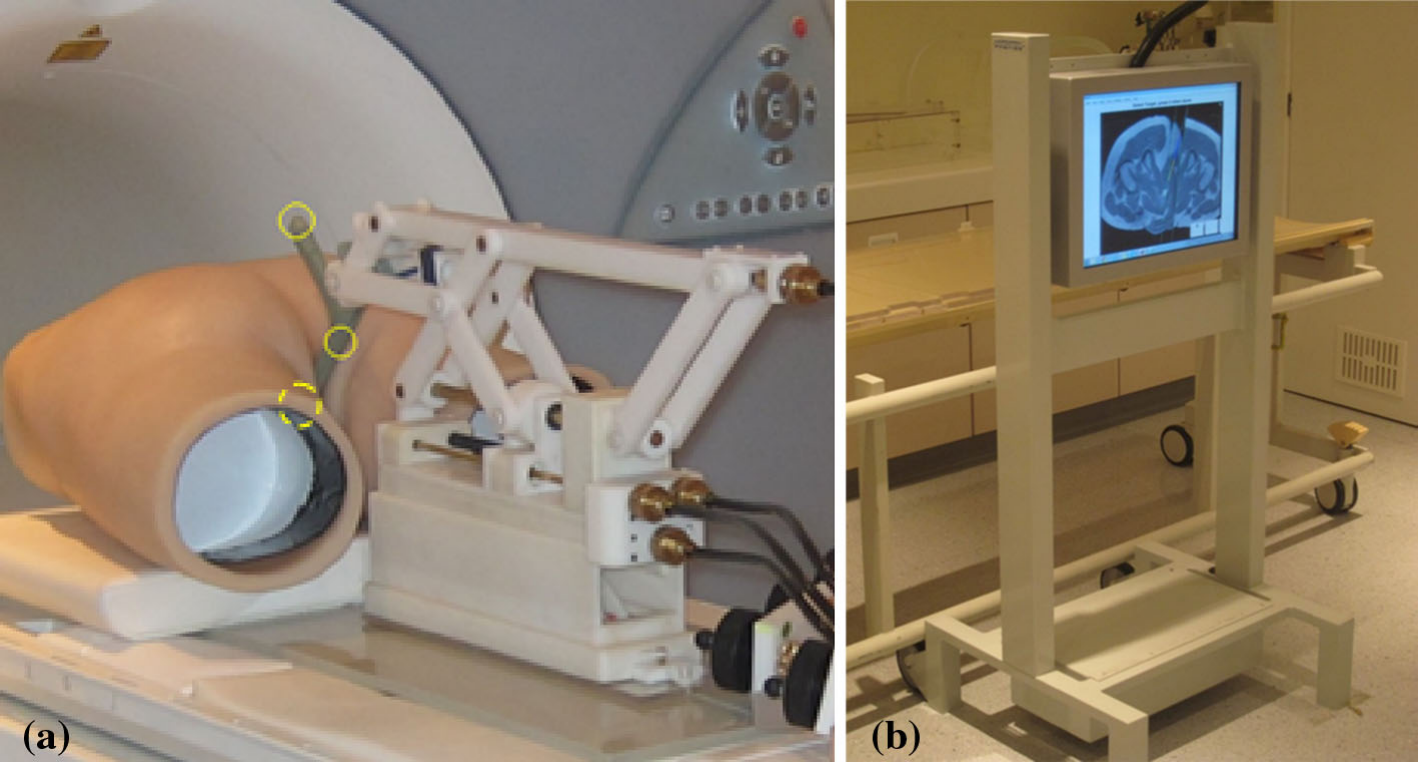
a MRI-guided prostate biopsy system; yellow circles indicate positions of the markers, b screen in a scanner room
